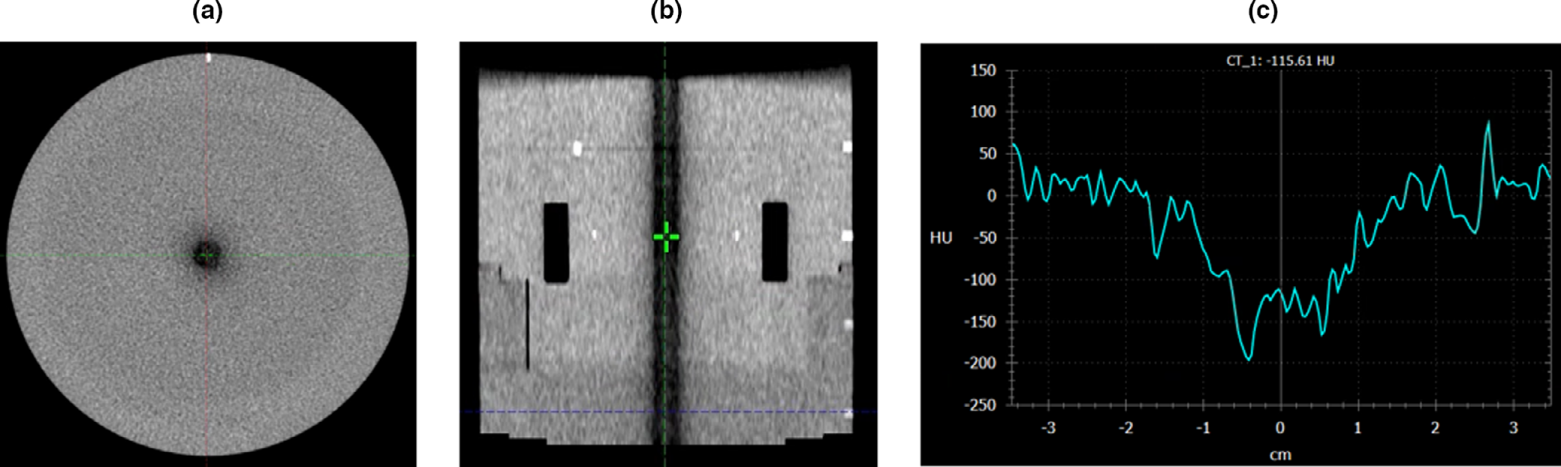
(a) Transverse and (b) sagittal planes of the module used for uniformity analysis of a cone‐beam computed tomography acquired using the Head technique and with the presence of a central dark artifact. (c) An HU line profile across the center of the transverse plane.
